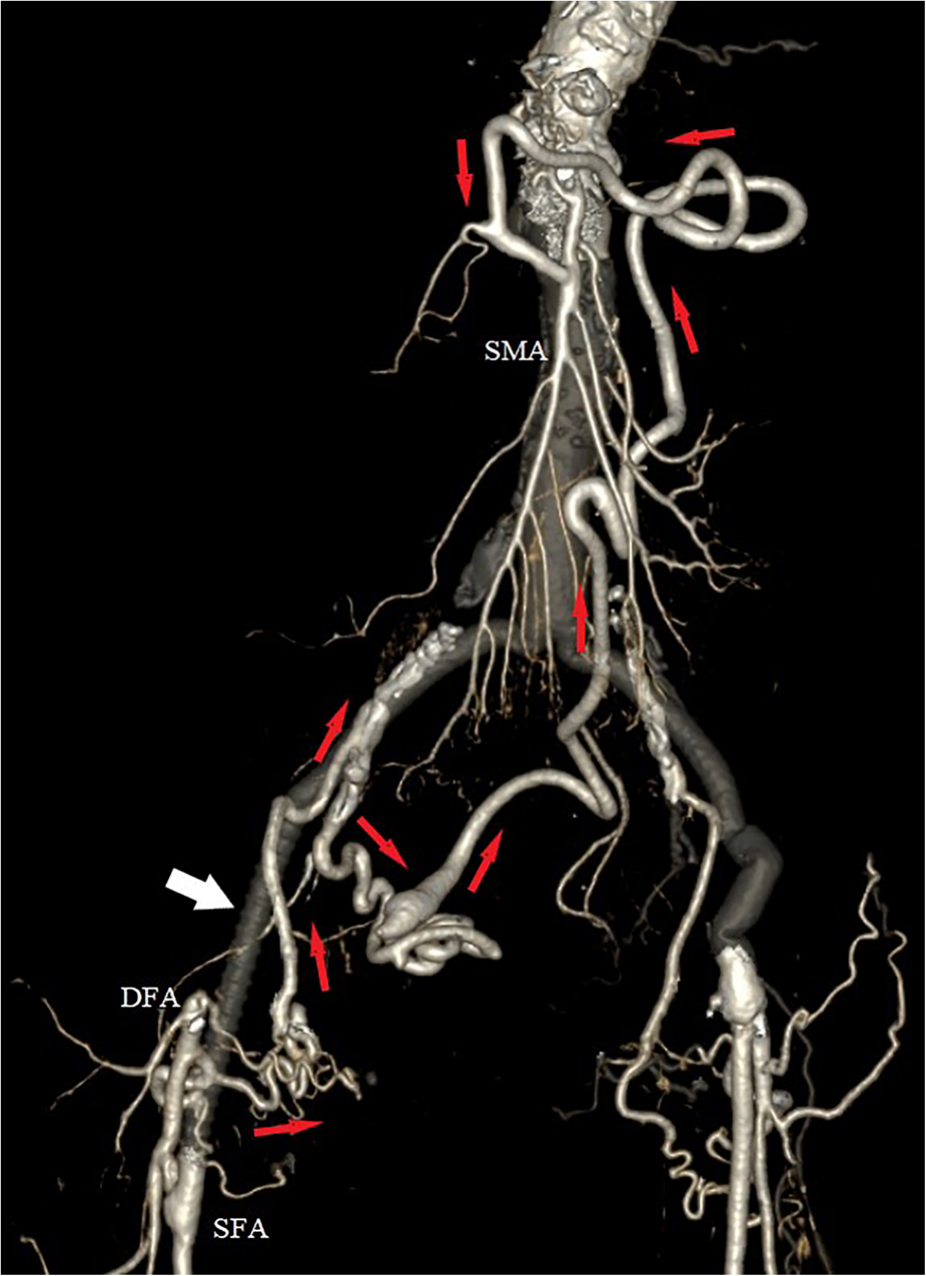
Postoperative 3D volume rendered computed tomography angiography (CTA), with exclusion of the aneurysm and preservation of the collateral network (red arrows) between the right deep femoral artery (DFA) and the superior mesenteric artery (SMA). White arrow indicates the interposition of the PTFE iliofemoral graft.
